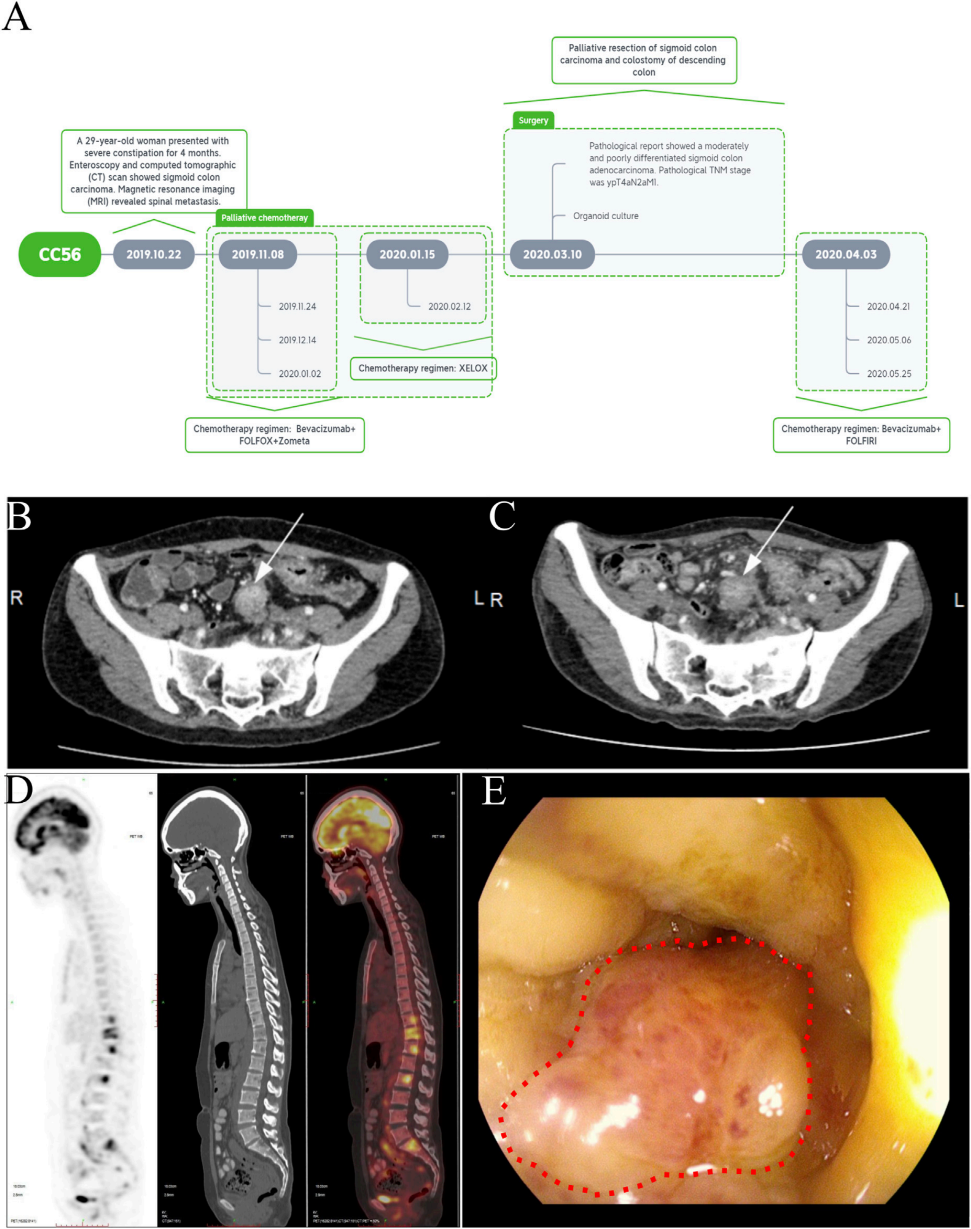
Treatment timeline and clinical characteristics of a case of primary advanced sigmoid colon cancer with bone metastasis. (A) Treatment timeline B-C. Abdominal computed tomography showing sigmoid colon carcinoma before (B) and after treatment (C) (white arrow). (D) PET-CT showing the multiple spinal metastases. (E) Enteroscopic image of stenosis caused by sigmoid colon carcinoma (red dotted circle).
